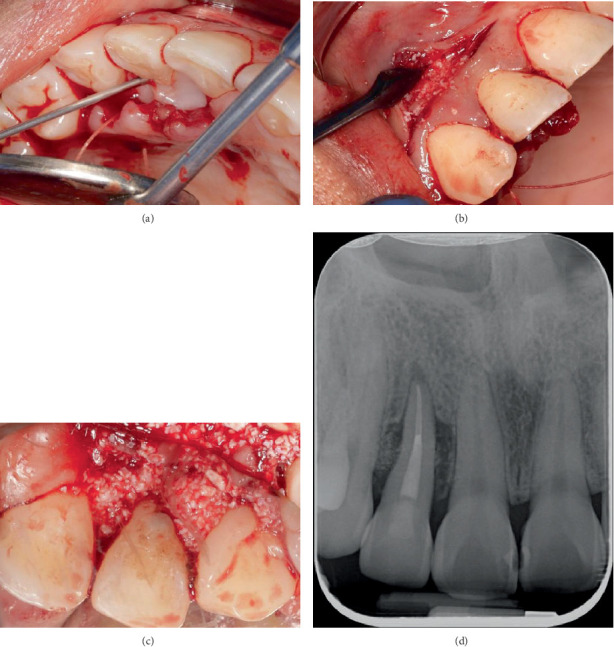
(a) Application of Straumann PrefGel in intrabony defect. Application of xenograft with EMD in (b) buccal aspect and (c) palatal aspect. (d) Periapical radiograph with Bio-Oss/EMD filling intrabony defect.
